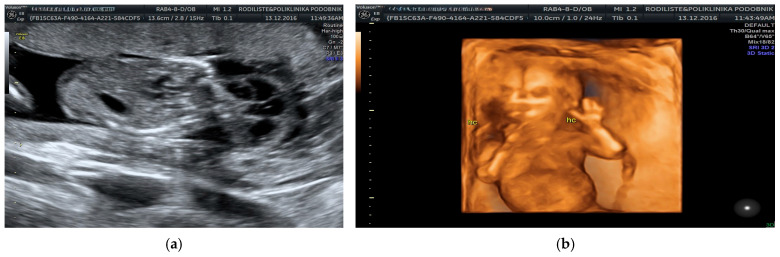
Transvaginal sonographic scan showing bilateral septated cystic hygroma in a fetus at 12.4 weeks of gestation. (a) Three-dimensional transvaginal ultrasound image of the same fetus. (b) Cytogenetic analysis revealed trisomy 21.
